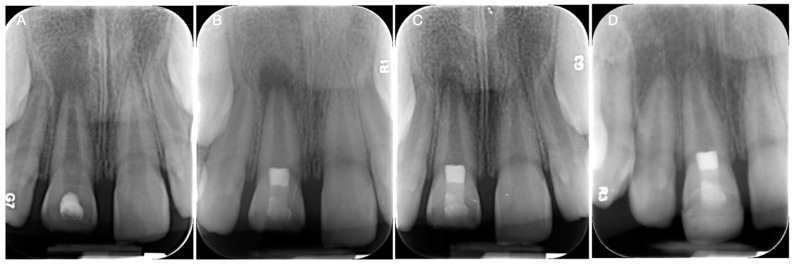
Clinical case using dHACMs for pulp regeneration. (A) Preoperative radiograph showing the maxillary right central incisor with a periapical lesion. The tooth was diagnosed with previously initiated therapy and symptomatic apical periodontitis (B) Postoperative radiograph after root canal disinfection, placement of dHACMs, and restoration. (C) Three-month follow-up showing a reduction in size of the periapical lesion. (D) Fourteen-month follow-up showing the complete resolution of the periapical lesion and the complete root formation.
